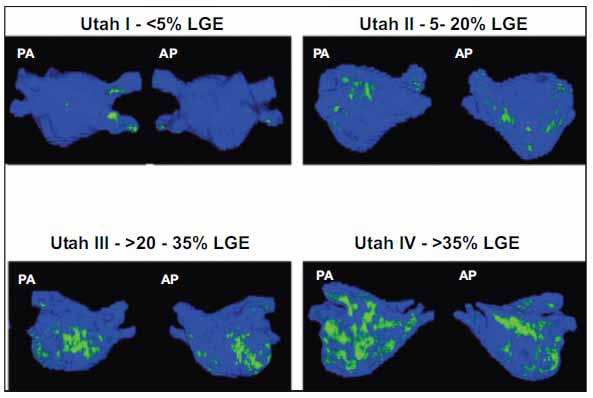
University of Utah Atrial Fibrillation LGE-MRI-based staging system. Utah I ≤ 5% atrial enhancement, Utah II >5–20%, Utah III > 20–35%, and Utah IV > 35%. The post-AF ablation recurrence rate was proportional to the amount of pre-AF ablation LA wall enhancement: there were no recurrences in stage 1, there was a 28% recurrence rate in stage 2 patients, a 35% recurrence in stage 3, and a greater than 50% recurrence in those patients with stage 4.
